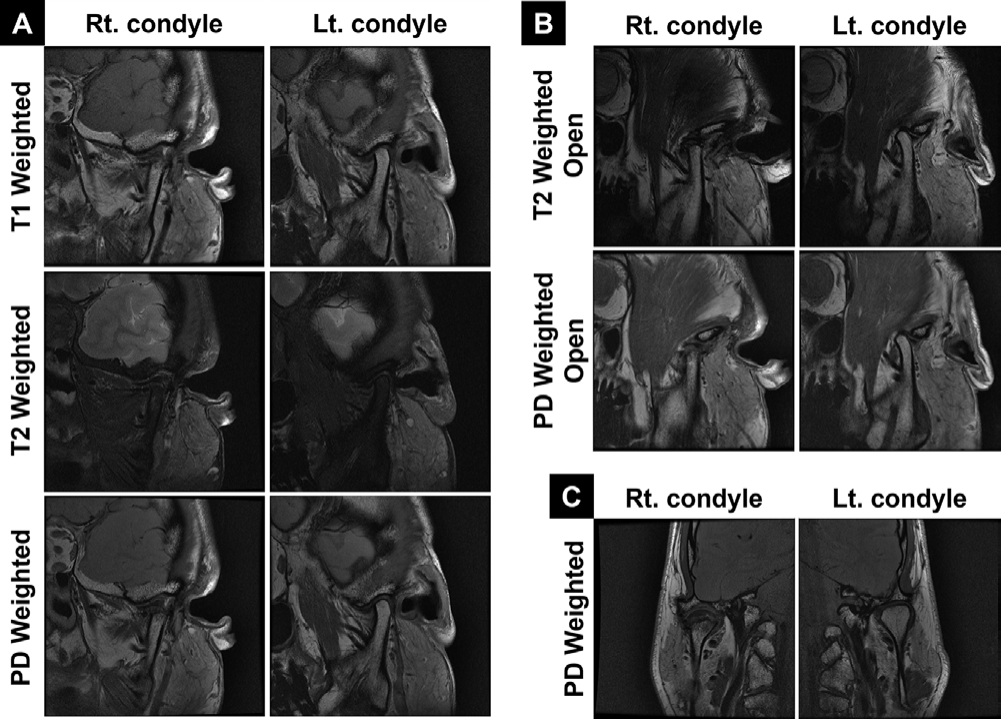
Six months postoperative follow-up MRI. (A) T1, T2, and PD-weighted oblique sagittal view in the closed-mouth position, showing resolution of the joint effusion. (B) T2 and PD-weighted oblique sagittal view in the open-mouth position, showing that the cortical bone at the upper end of the right condyle neck is not yet clear, with no signs of adhesion or ankylosis of the articular disc. (C) PD-weighted coronal view in the closed-mouth position. MRI = magnetic resonance imaging.
